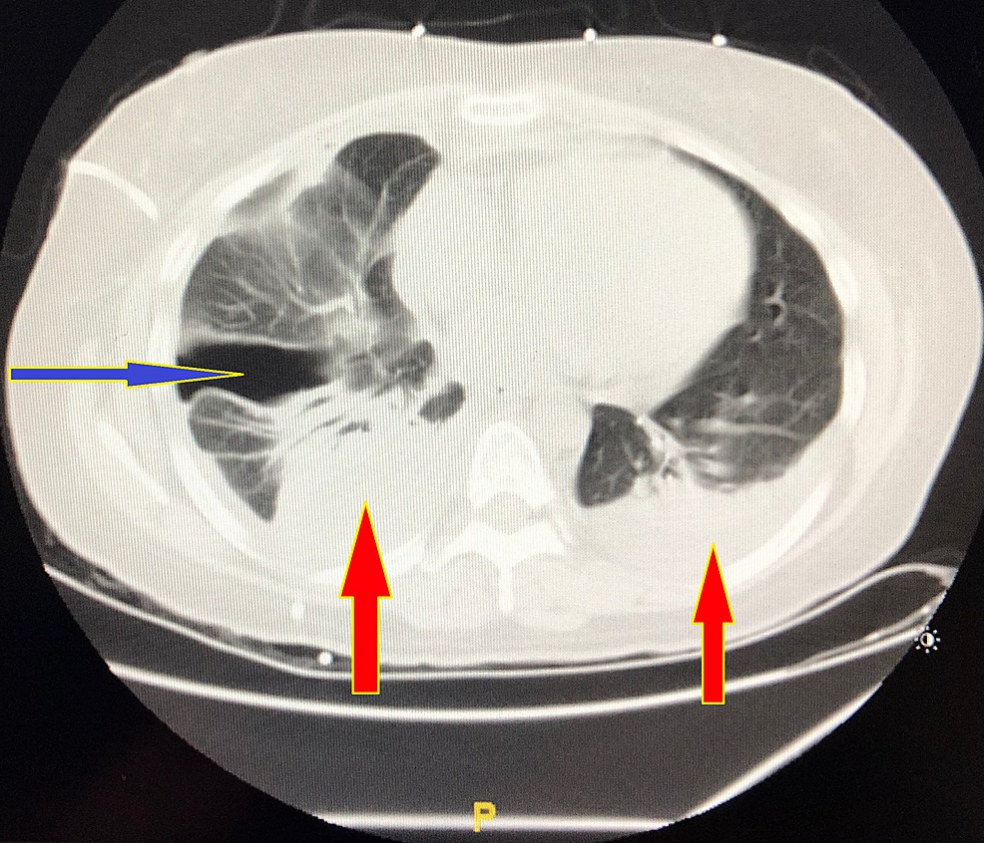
CT chest without contrast showing right-sided small encysted pleural effusion (red arrow on the right) with pneumothorax (blue arrow), and small, free, left-sided pleural effusion (red arrow on the left).
